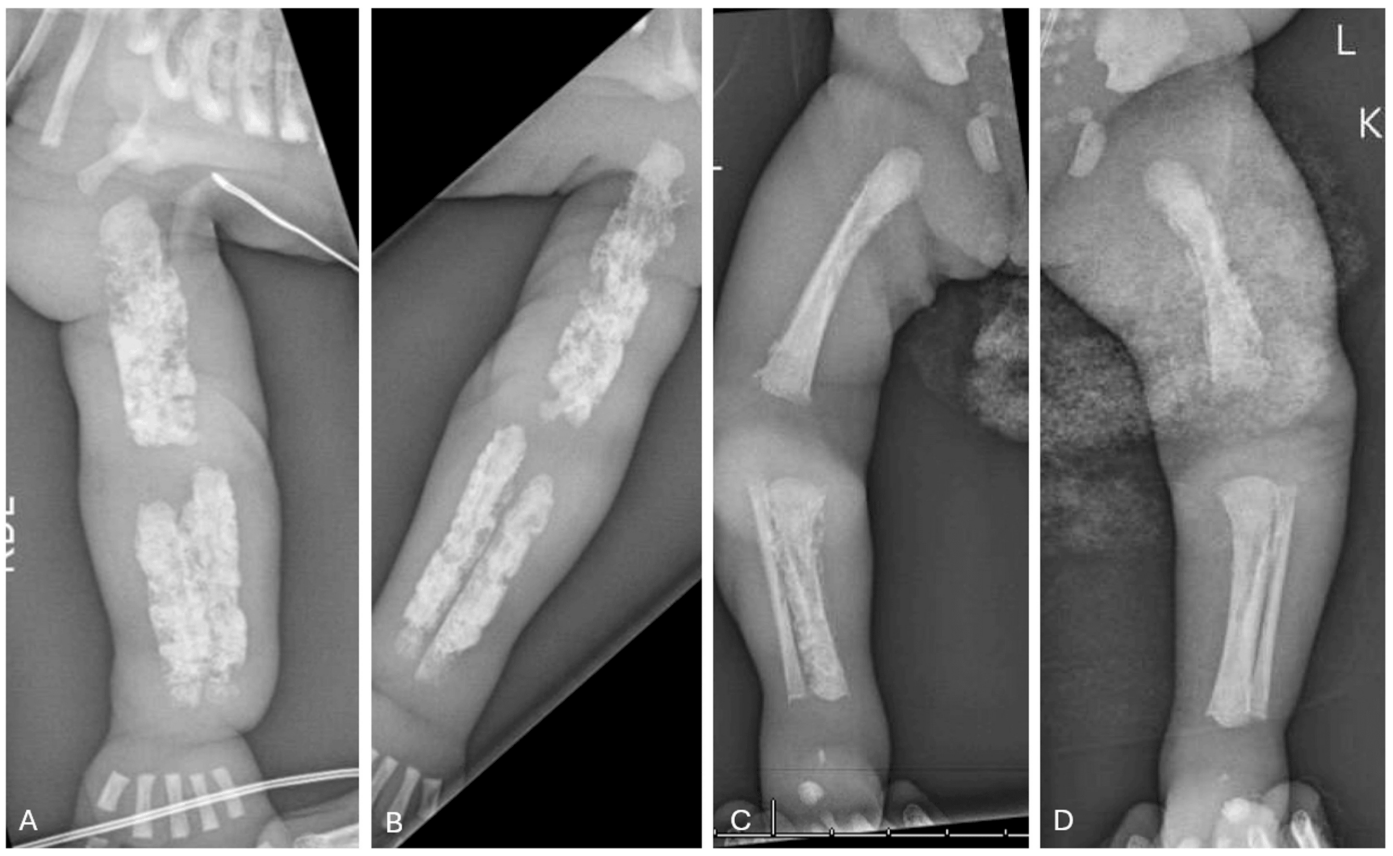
Radiographs of right (A) and left (B) humeri as well as right (C) and left (D) femora at birth. Radiographs demonstrate diffuse, permeative "cotton wool" appearance of the long bones as well as the partially-imaged ribs.
